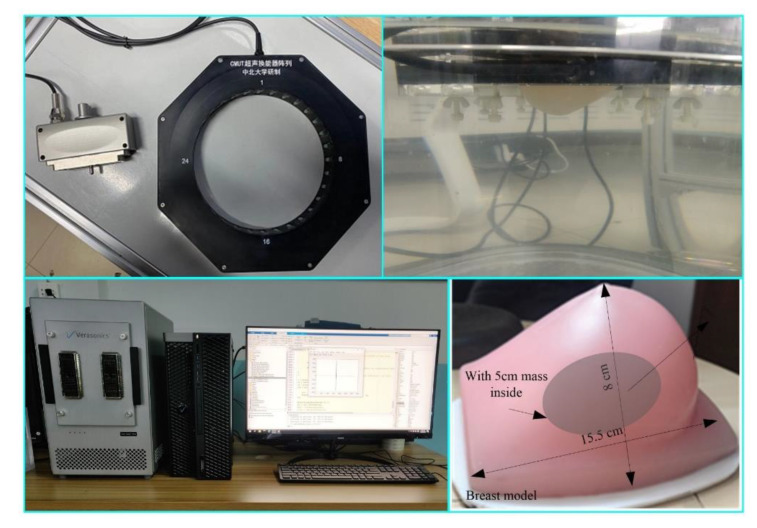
Experimental platform of breast ultrasound tomography system based on a CMUT ring array.
